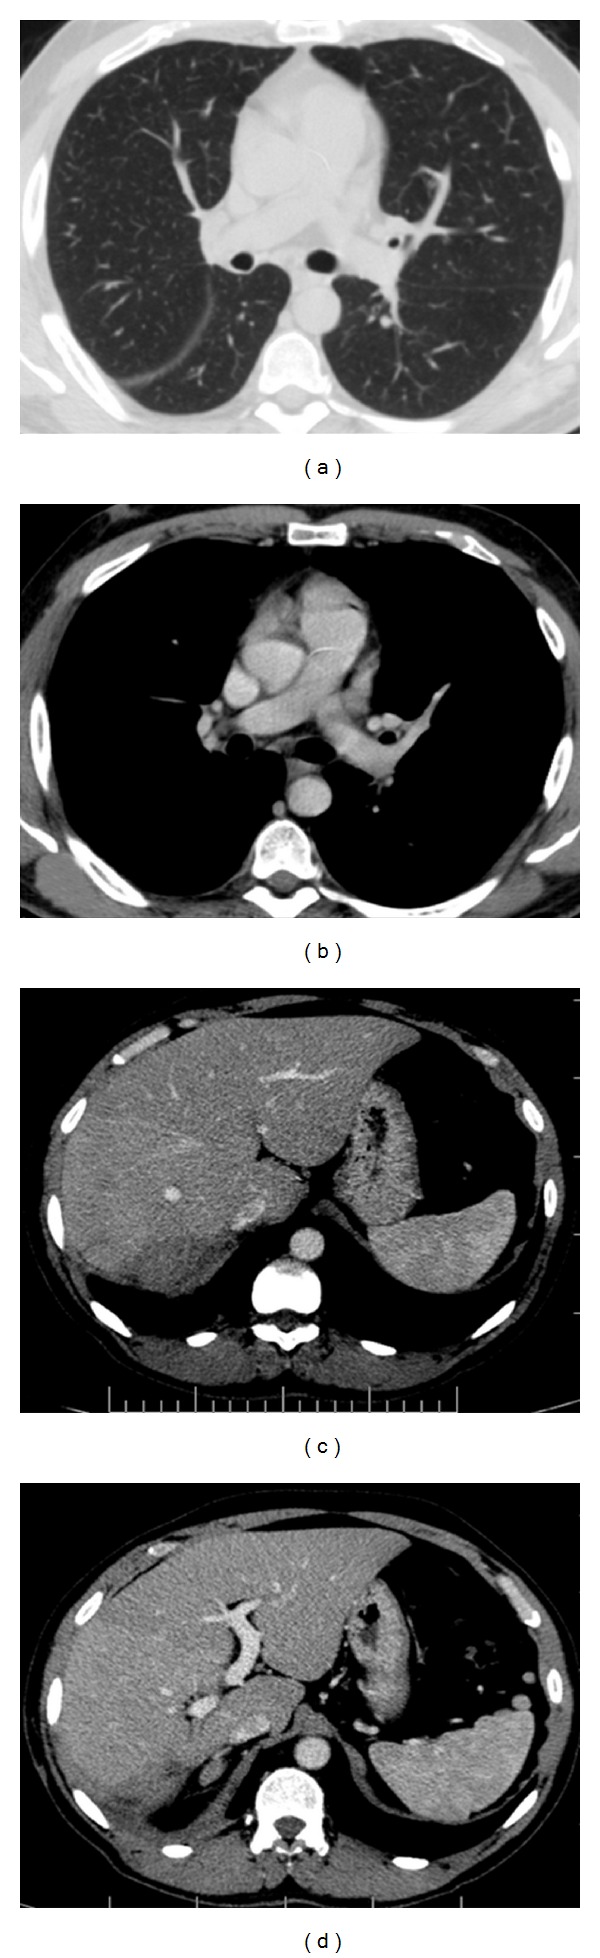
(a) and (b) computed tomography showing complete recovery of pulmonary lesions in right hemithorax; (c) and (d) almost complete disappearance of hepatic lesion (arrows).
